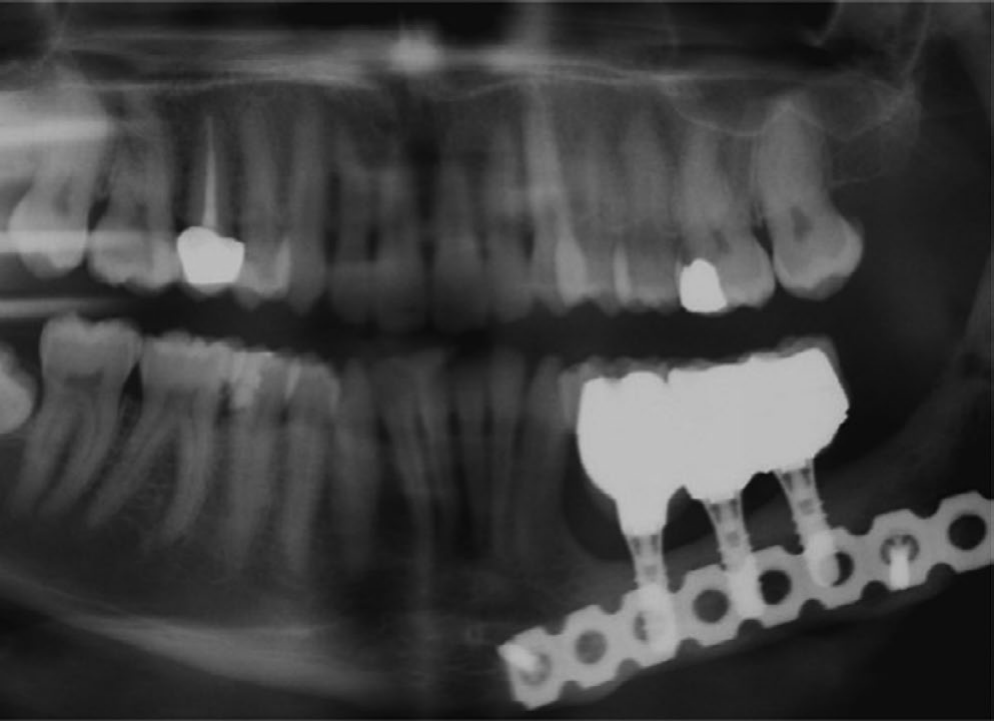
Radiograph after 3 years follow‐up
